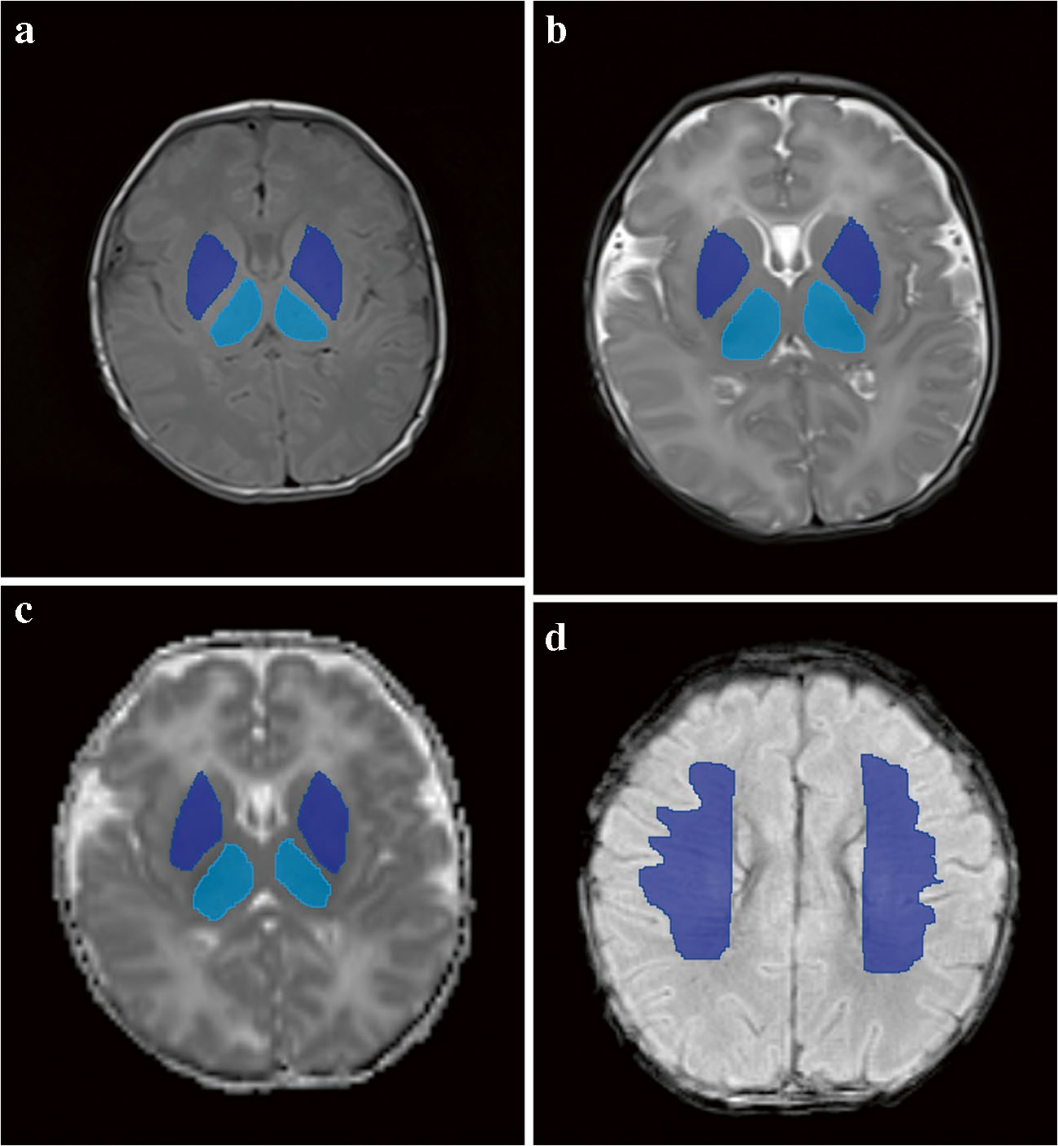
Radionics analysis on axial magnetic resonance images in a 37-week-old male neonate with mild hypoxic ischemic encephalopathy. a–c Regions of interest were placed on the basal ganglia (*dark blue*) and thalami (*light blue*) on the axial T1-weighted (W) images (a), T2-W images (b) and apparent diffusion maps (c). d Susceptibility-weighted image with regions of interest placed on the deep medullary veins (*dark blue*)
